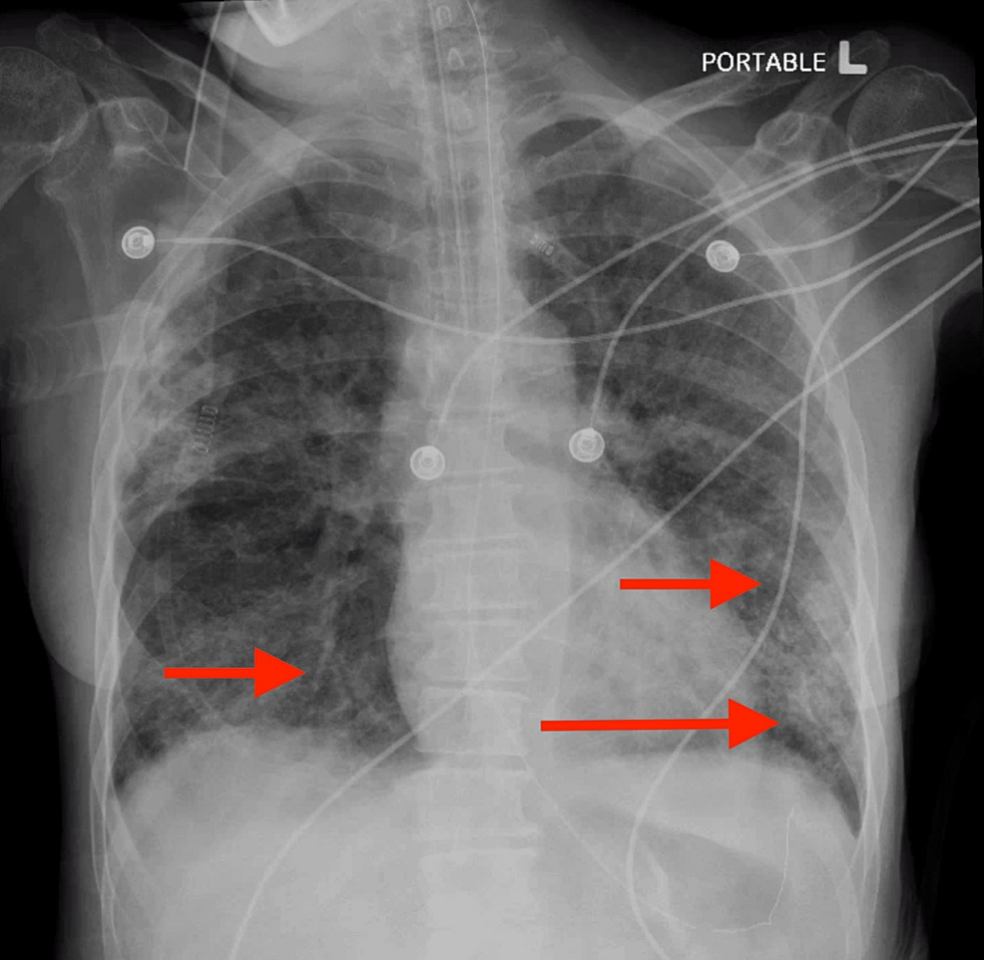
X-ray chest showing patchy opacities (red arrows).
